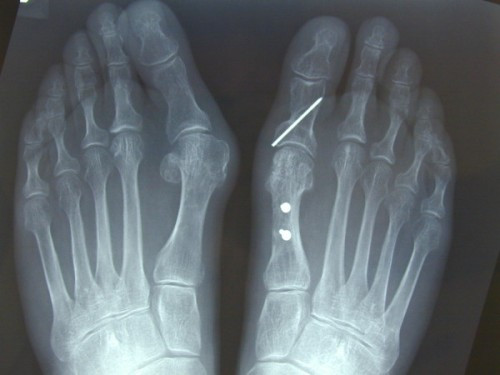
Postoperative x-ray of the rotation scarf and Akin osteotomies in the right foot. This x-ray demonstrates fixation of the osteotomy, realignment of the sesamoids and reduction of the intermetatarsal and hallux valgus angle while preserving the length of the metatarsal.
